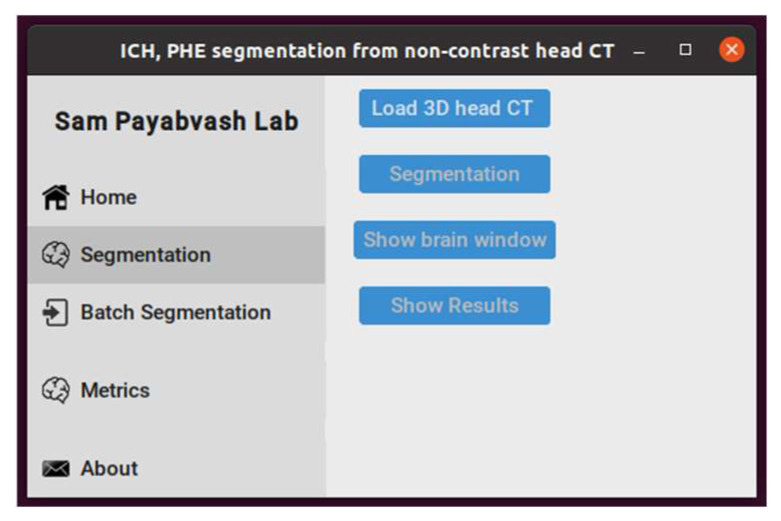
The interface of GUI application for hematoma and edema segmentation from non-contrast head CT.
